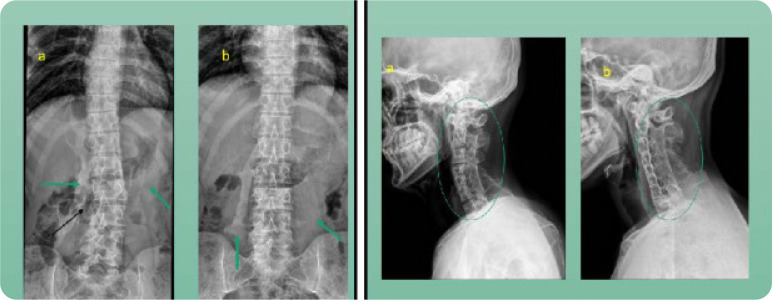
Heterotopic ossification seen in proband A and B on Lumbar AP view radiographs on the left. Lumbar scoliosis (black arrow) shown in Proband A. Loss of normal cervical lordosis, bridging ossification of posterior elements seen in both patients on the right.
